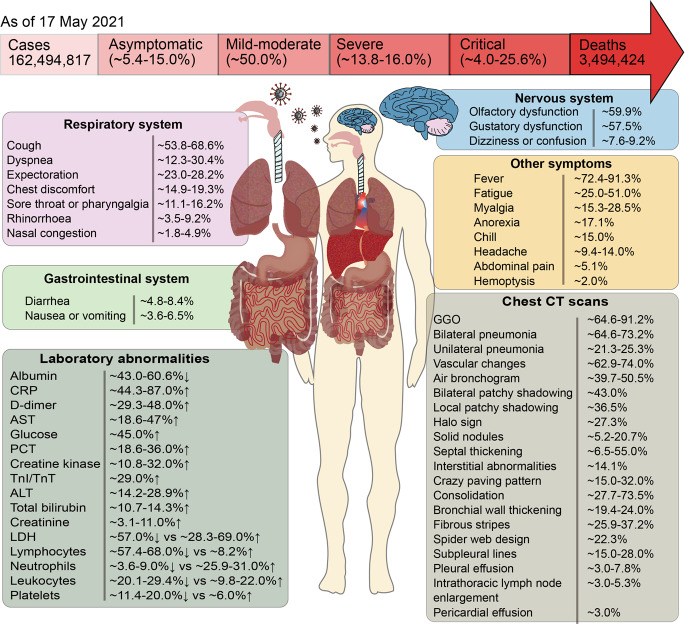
Overview of clinical characteristics of COVID-19. CRP C-reactive protein, AST aspartate aminotransferase, PCT procalcitonin, TnI/TnT troponin I/troponin T, ALT alanine aminotransferase, LDH lactate dehydrogenase, CT computed tomography, GGO ground-glass opacity
